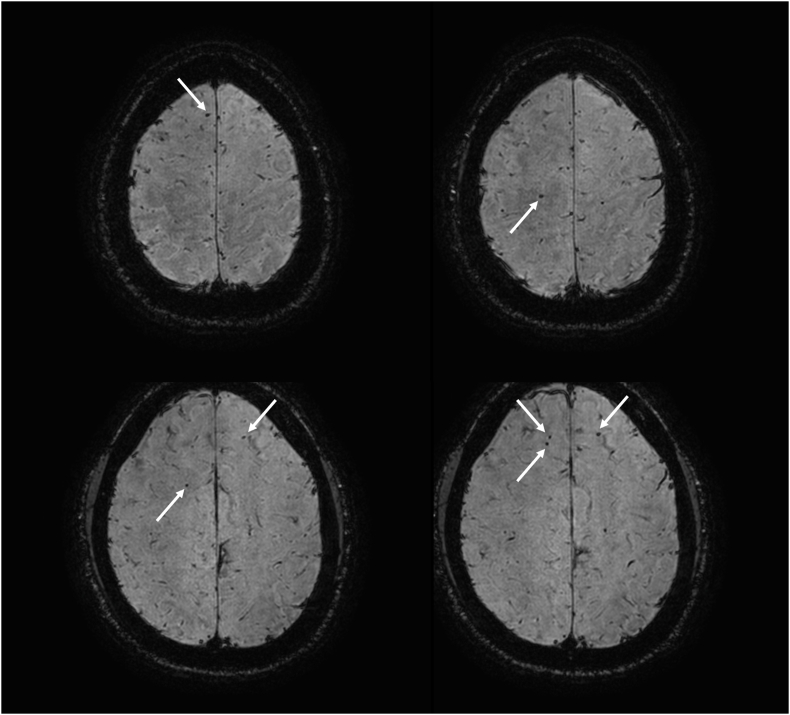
Suspected case of cerebral fat embolism (CFE) in a 38-year-old man who was injured due to a fall and diagnosed with right acetabular fracture and right kidney injury. The diagnostic criteria for fat embolism syndrome (FES) were not applicable, and there were no neurological problems. A brain magnetic resonance imaging (MRI) was performed on day 16. There were many low-intensity signs (white arrow), indicating small hemorrhages in the bilateral white matter of the frontal lobe on susceptibility-weighted imaging (SWI), which was assessed as diffuse axonal injury or CFE.
